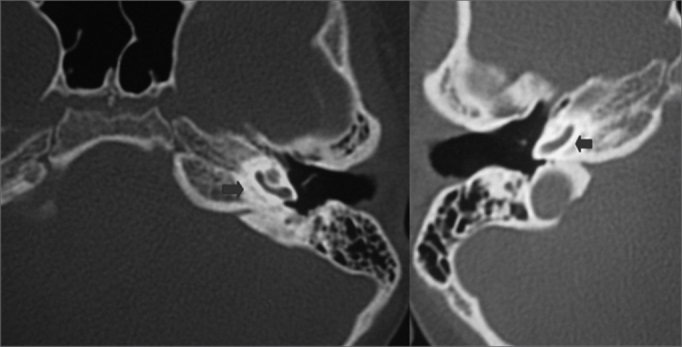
Computed tomography (CT) of the temporal bones; axial slices along the round window showing pericochlear hypodensity (otosclerosis) (blue arrows).
